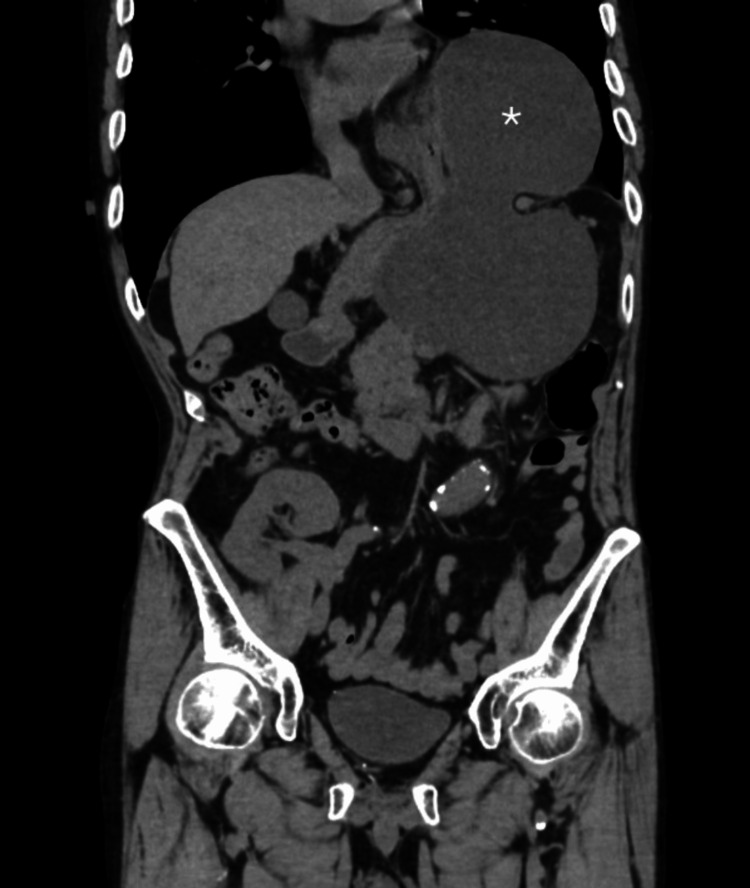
CT demonstrating an incarcerated hiatal hernia Coronal CT on admission demonstrating an incarcerated hiatal hernia with herniation. The asterisk (*) indicates the herniated stomach in the left thoracic cavity.
